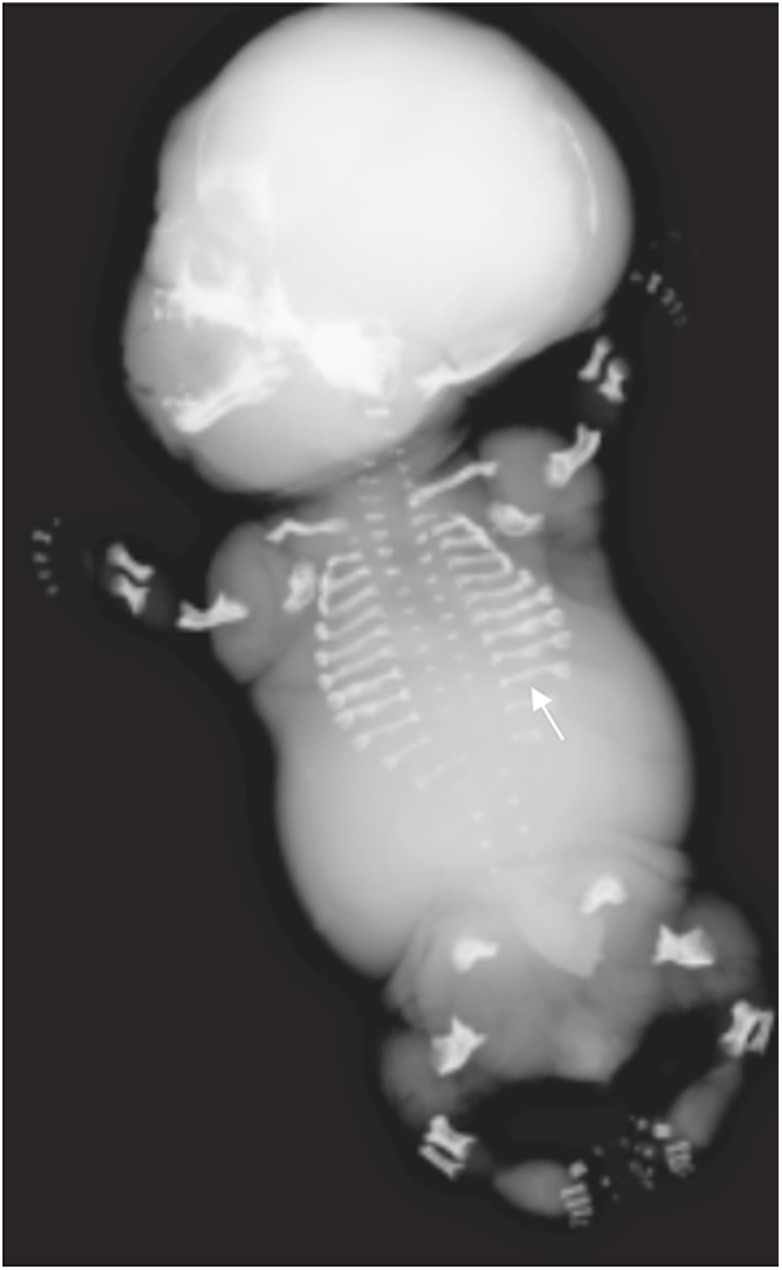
Radiographic features in a female fetus with achondrogenesis type IA*.* Anteroposterior radiograph in the most severe form of the disease shows short limbs, short ribs, deficient ossification of the pelvis and skull, and “beaded” ribs due to healing fractures (*arrow*). [Image reproduced with permission from Radcliffe Publishing [38], page 215, case 3, image 3b]
